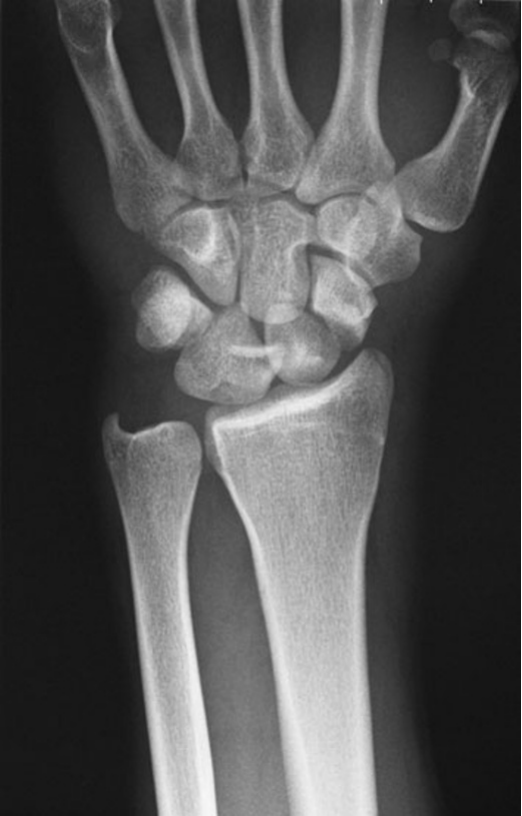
Wrist PA X-ray at presentation showing a displaced scaphoid fracture with disruption of Gilula’s arcs (1 and 2)
